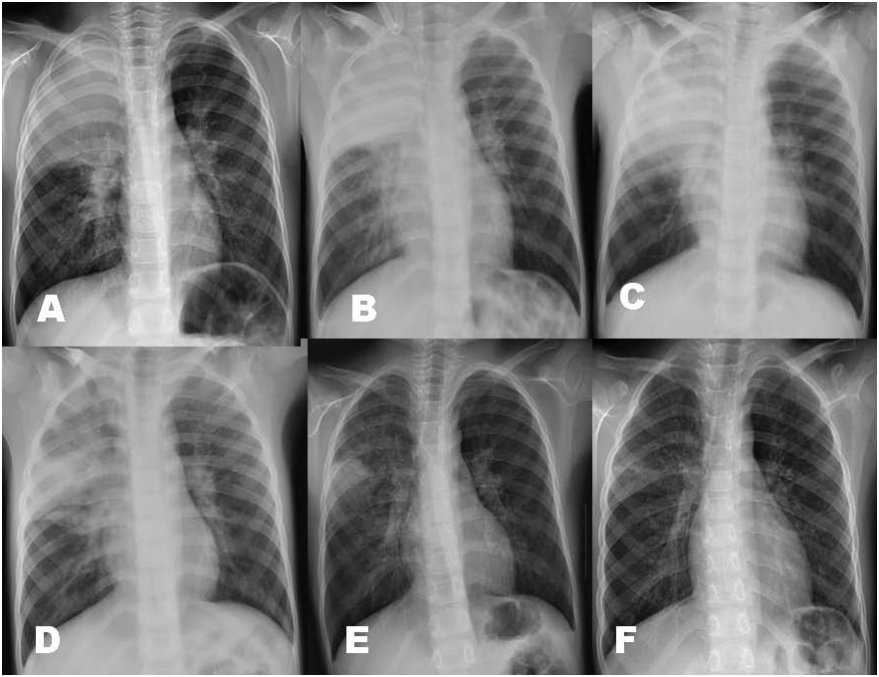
The chest radiographs of a patient who were treated with MP and intravenous immunoglobulin (IVIG) (male, 7-year old); on admission (Figure 5-A), before MP treatment (Figure 5-B), after 2 doses of MP infusion (Figure 5-C), just after termination of IVIG infusion (Figure 5-D), 8 h after IVIG infusion (Figure 5-E), and 3 days after MP and IVIG treatment (Figure 5-F).
